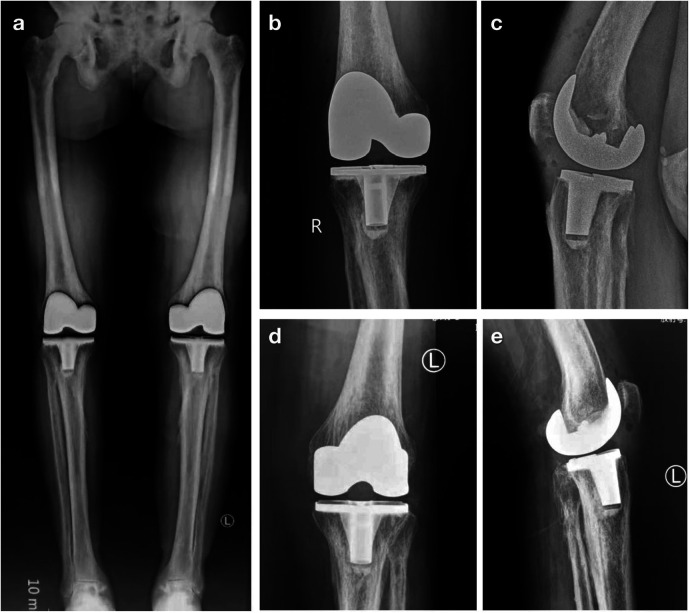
Postoperative radiographic appearance. (a) The full-length lower limb anteroposterior radiographic view shows restored lower limb alignment, with the right knee (b) anteroposterior and (c) lateral views and left knee (d) anteroposterior and (e) lateral views showing the excellent positioning, alignment, and angle of the prostheses.
